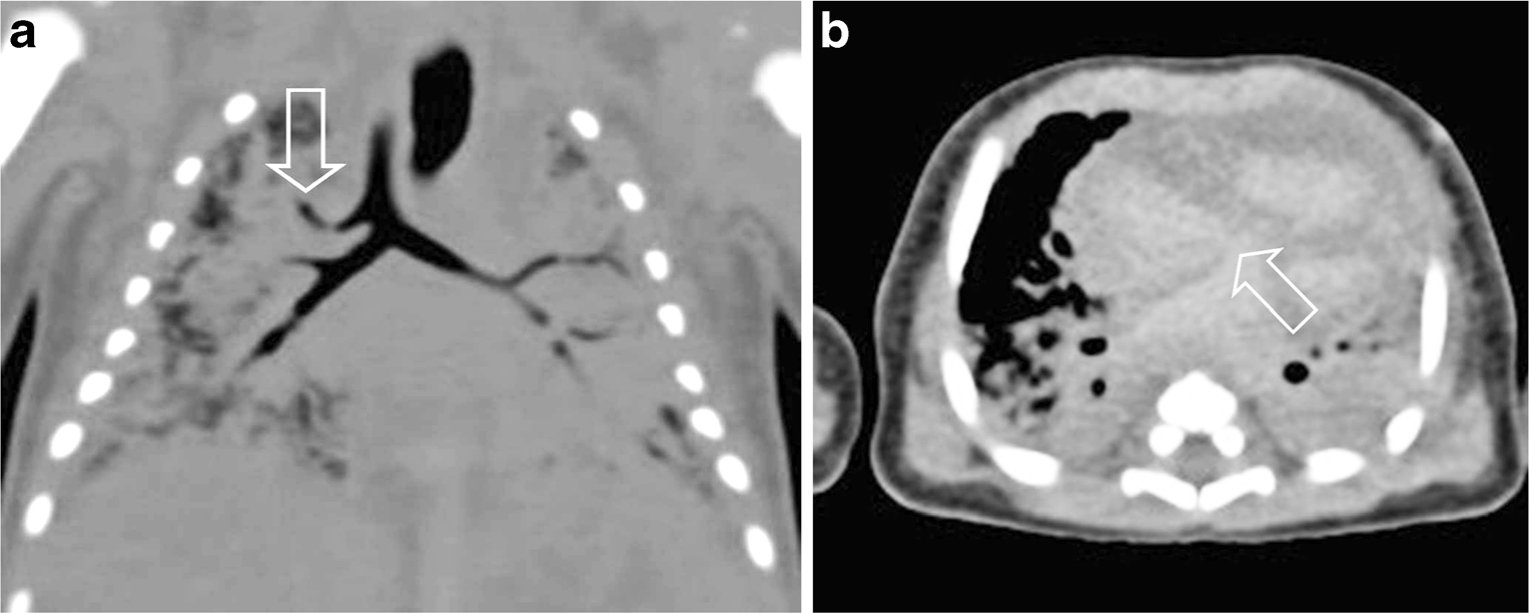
Cardiovascular cause of death. Postmortem CT in a 5-day-old boy. a Coronal reconstruction shows a tracheal right upper lobe bronchus (*arrow*). b Axial image shows an atrial septal defect (*arrow*). Both findings were confirmed at autopsy
